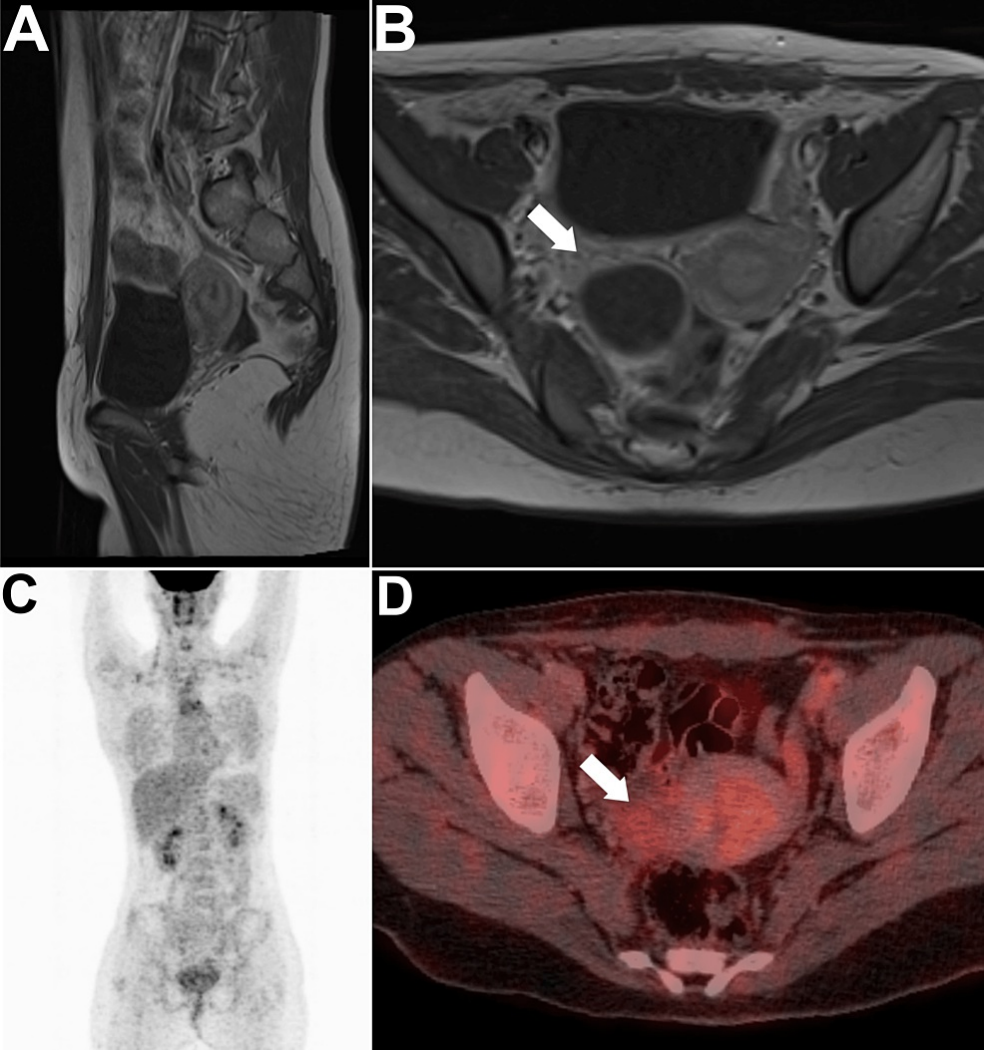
Diagnostic scans at the end of the third year following surgery (A) Sagittal T1 pelvic MRI revealed a clear surgical bed that was unremarkably normal. (B) Axial T1 MRI was also unremarkable. (C) Maximum intensity projection image rendered a normal scan. (D) Axial PET/CT image of the pelvis revealed a clear tumor bed that was unremarkably normal (arrows).
